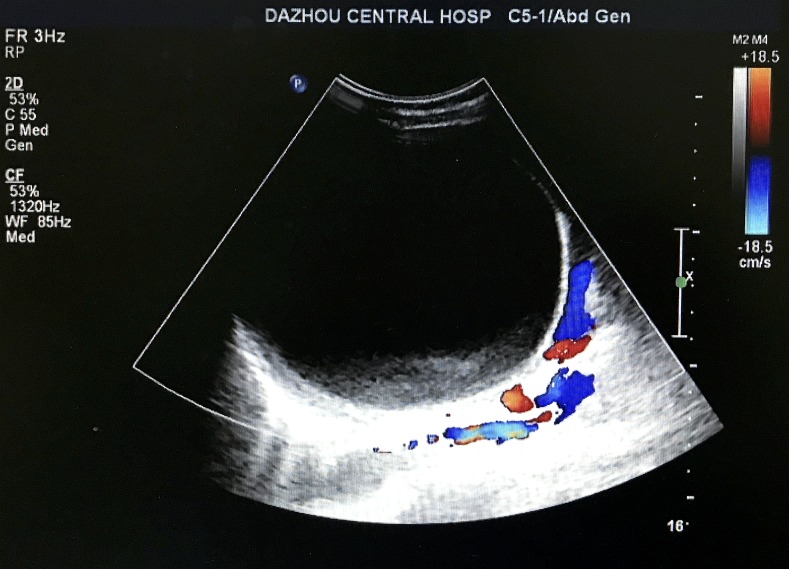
Abdominal ultrasonography showing a 13.5 × 12.1-cm–sized anechoic solitary, thin-wall cyst in the splenic parenchyma. This figure appears in color at www.ajtmh.org.
